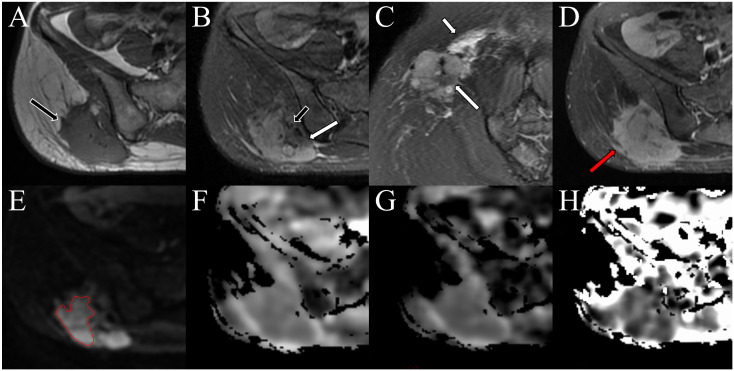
A 39-year-old patient with fibrosarcoma of the right buttock. (A) Axial T1-weighted image showed an irregular and slightly hyperintense mass (long black arrow). (B, C) Axial and coronal T2-weighted images showed a heterogeneous mass exhibiting internal necrosis, band sign (long white arrow), and flow void (short black arrow), accompanied by peritumoral edema (short white arrow) in the adjacent fat layer. (D) Contrast-enhanced T1-weighted image showed heterogeneous enhancement of the lesion, invasion into the surrounding fat space, and presentation of fascial tail sign (long red arrow). (E) The solid portion of the lesion showed marked hyperintensity on the IVIM (b = 800 s/mm^2^) image; (F) the standard-ADC_mean_ and standard-ADC_min_ values were 1.13 × 10–^3^ mm^2^/s and 1.05 × 10–^3^ mm^2^/s; (G) the D_mean_ and D_min_ values were 0.91 × 10^-3^mm^2^/s and 0.72 × 10–^3^ mm^2^/s; and (H) the f_mean_ and f_min_ values were 12.90 and 7.81.
